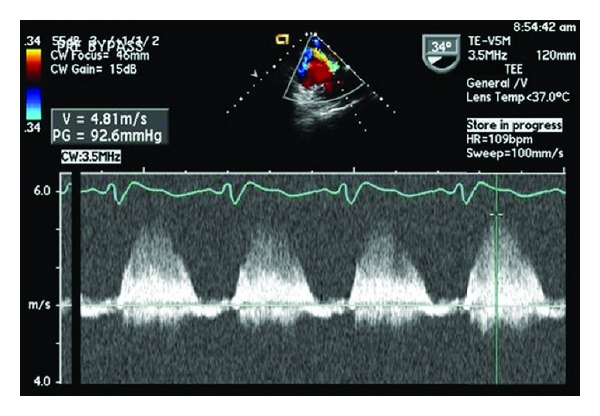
Transesophageal echocardiography: midesophageal 4 chamber view with emphasis in tricuspid valve at 34°. Continuous wave Doppler assessment of tricuspid valve regurgitation used to calculate right ventricular systolic pressure (RVSP). Note peak pressure gradient of 92 mm Hg.
